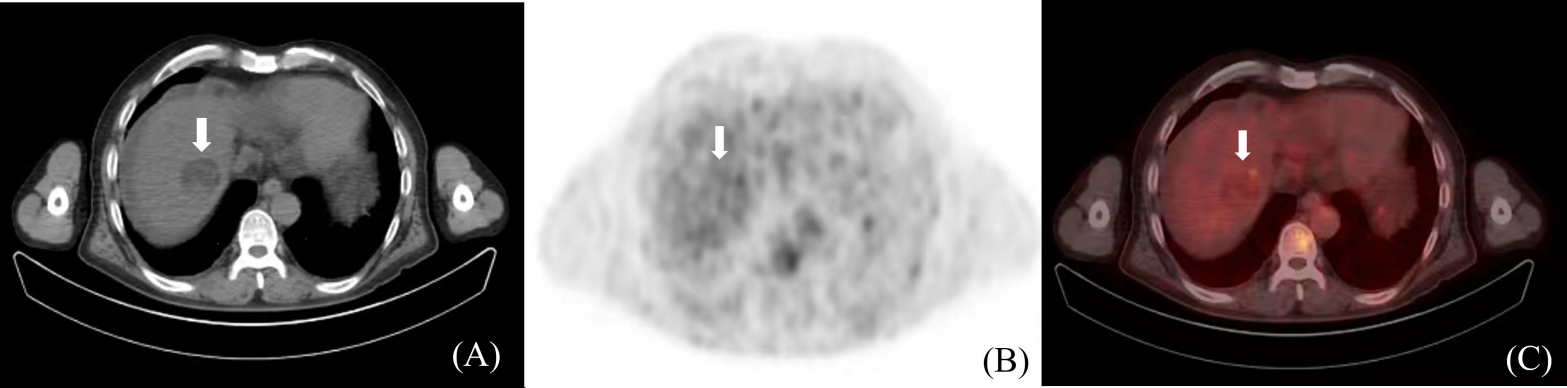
HCC PET/CT border imaging. (A) CT scan without contrast (B) PET images (C) PET/CT images.
